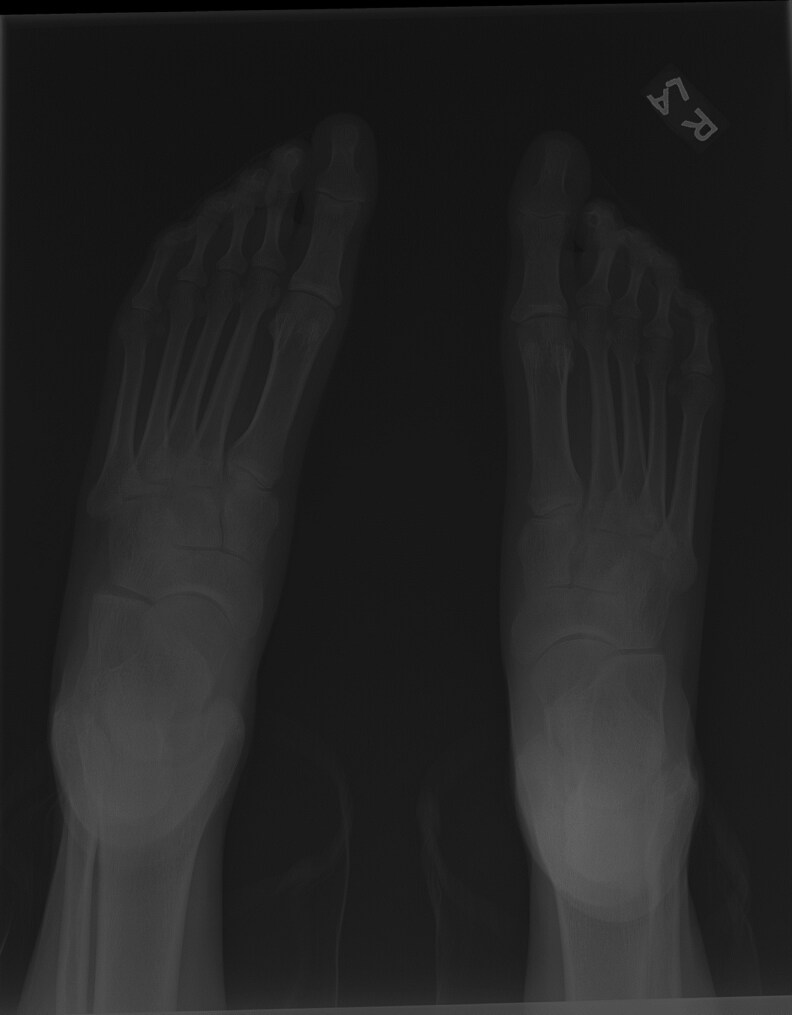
Arachnodactyly of feet on x-ray.
